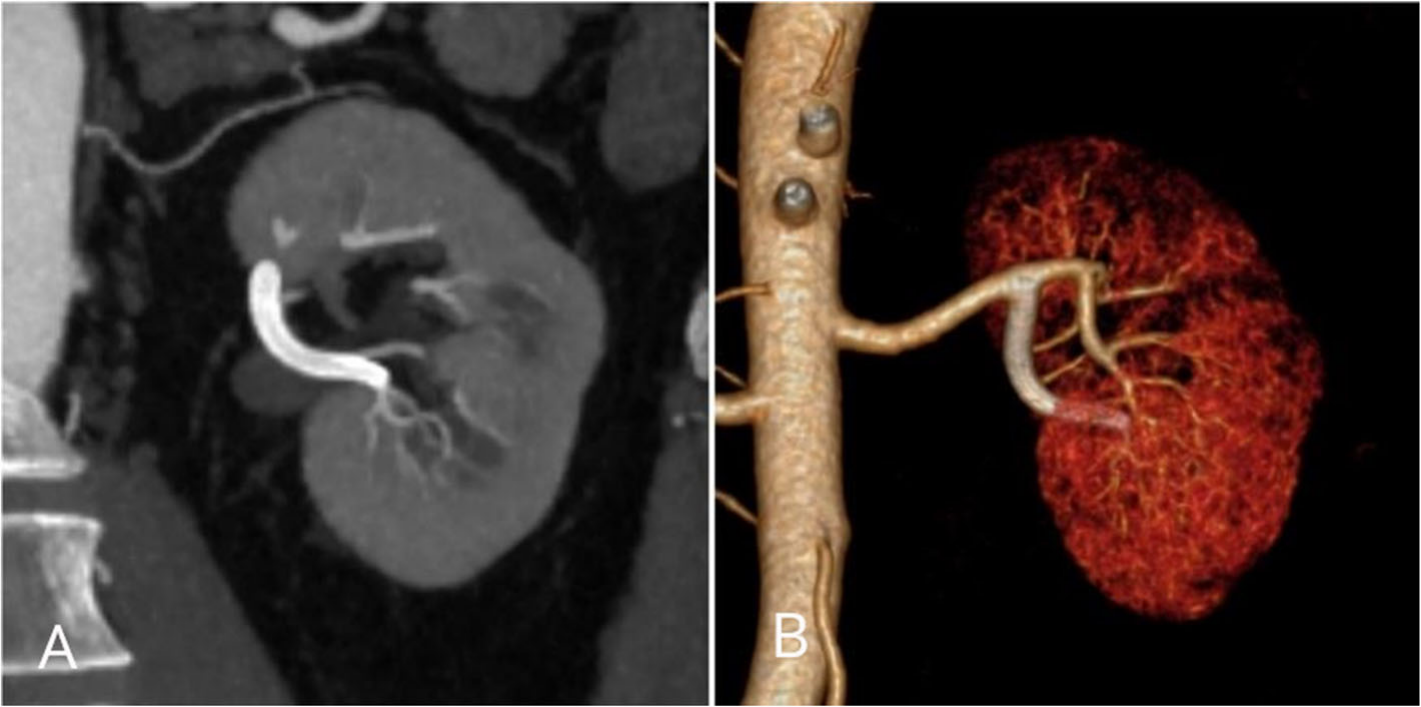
3-week follow up CTA. a) coronal MIP CTA and corresponding 3D volume rendered imaging demonstrating complete thrombosis and occlusion of the aneurysm without end organ or stent complication
